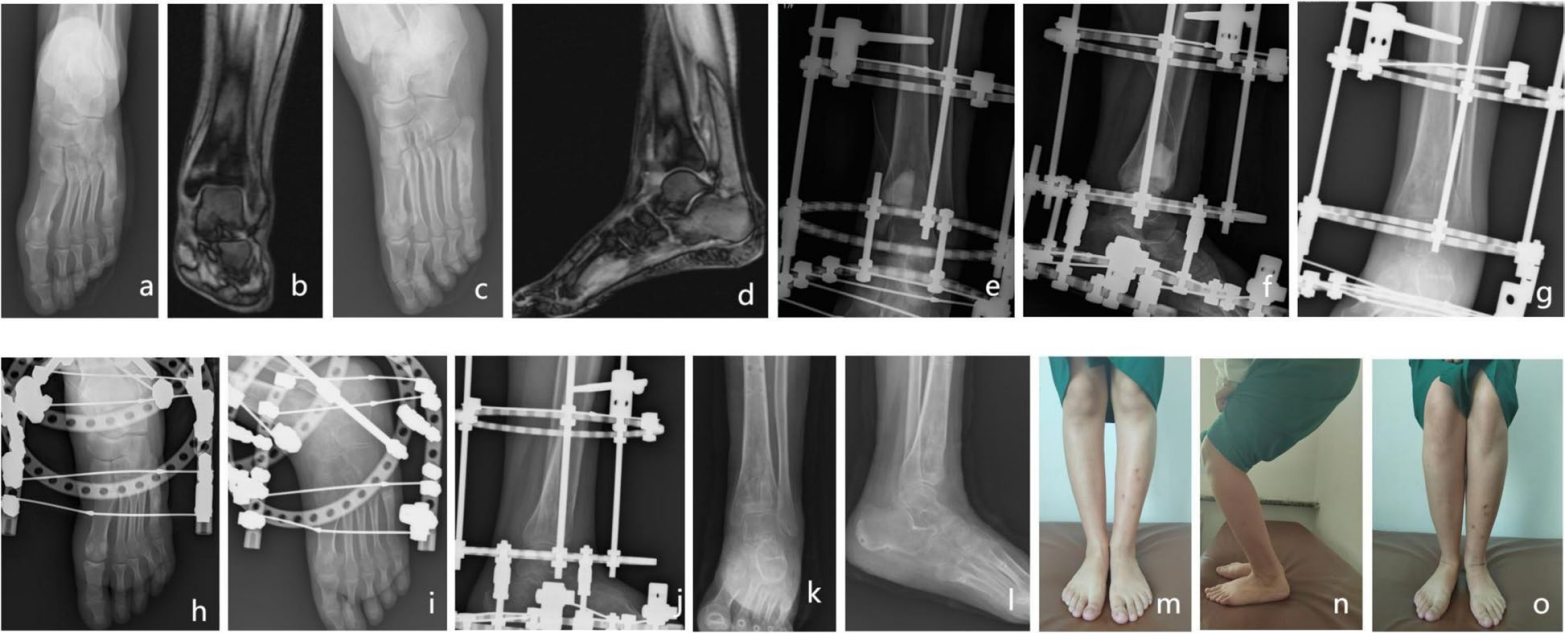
Patient, female, 17 years old, Osteomyelitis of the left metatarsal and left tibial bones with no apparent cause. a-d Preoperative X-ray and CT examination showed infection of the metatarsal and tibia; e–h Radical debridement, drug-loaded calcium sulfate implantation and external fixation showed anastomosis of the drug-loaded calcium sulfate with the bone defect site and stability of the external fixation frame on X-ray examination 1 day after surgery; i-j Good healing of the bone defect site on X-ray examination before removal of the external fixation frame 6 months after surgery; k-l Good surgical recovery on X-ray examination 7 months after surgery and 1 month after removal of the external fixation frame; m–n 24 months post-operative, full weight-bearing of the affected limb and almost normal gait
